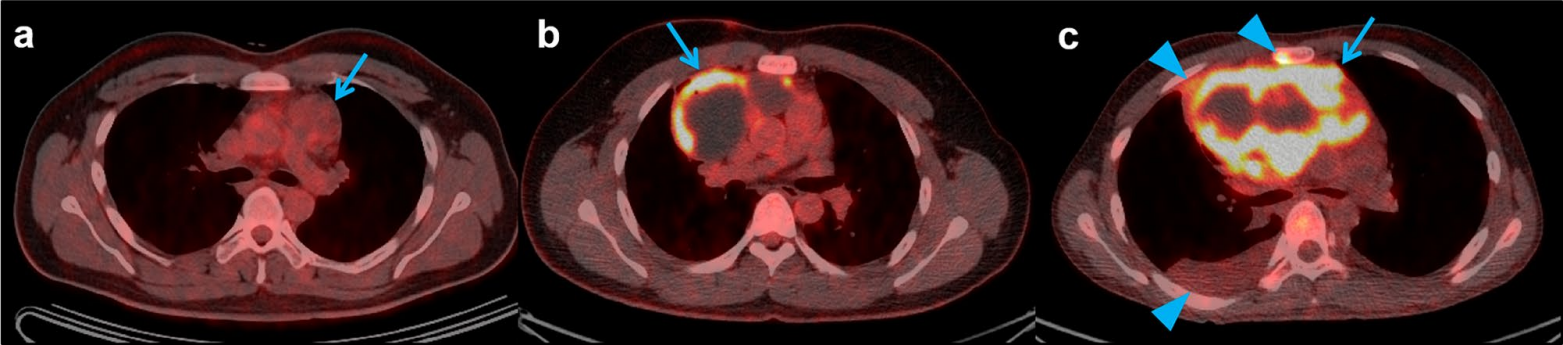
Representative ^18^F-FDG PET/CT cases of mediastinal germ cell tumors (MGCTs) with the pathologic subtype. (a) A 21-year-old man with benign MGCT (mature teratoma) showing grade 1 uptake, homogenous distribution, and round contour (SUVmax 1.9, arrow). (b) A 31-year-old man with seminoma showing grade 3 uptake, heterogenous distribution, and lobulated contour (SUVmax 15.0, arrow). (c) A 22-year-old man with yolk cell tumor showing grade 3 uptake, heterogenous distribution, and infiltrative contour (SUVmax 18.7, arrow) with anterior pleural invasion, pleural effusion, and bone metastasis in the sternum (arrowheads).
